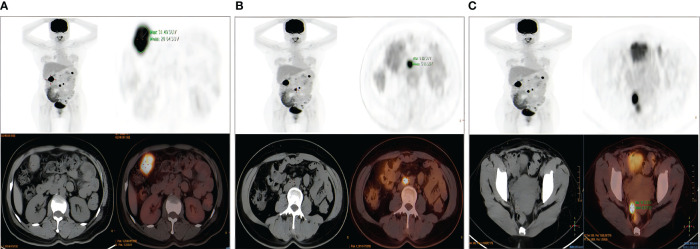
Positron emission tomography–computed tomography. (A) Nodular lesion anterior to the hepatic flexure of colon. (B) Enlarged retroperitoneal abdominal para–aortic lymph nodes. (C) Multiple soft–tissue masses and nodules on the right wall of the pelvis.
